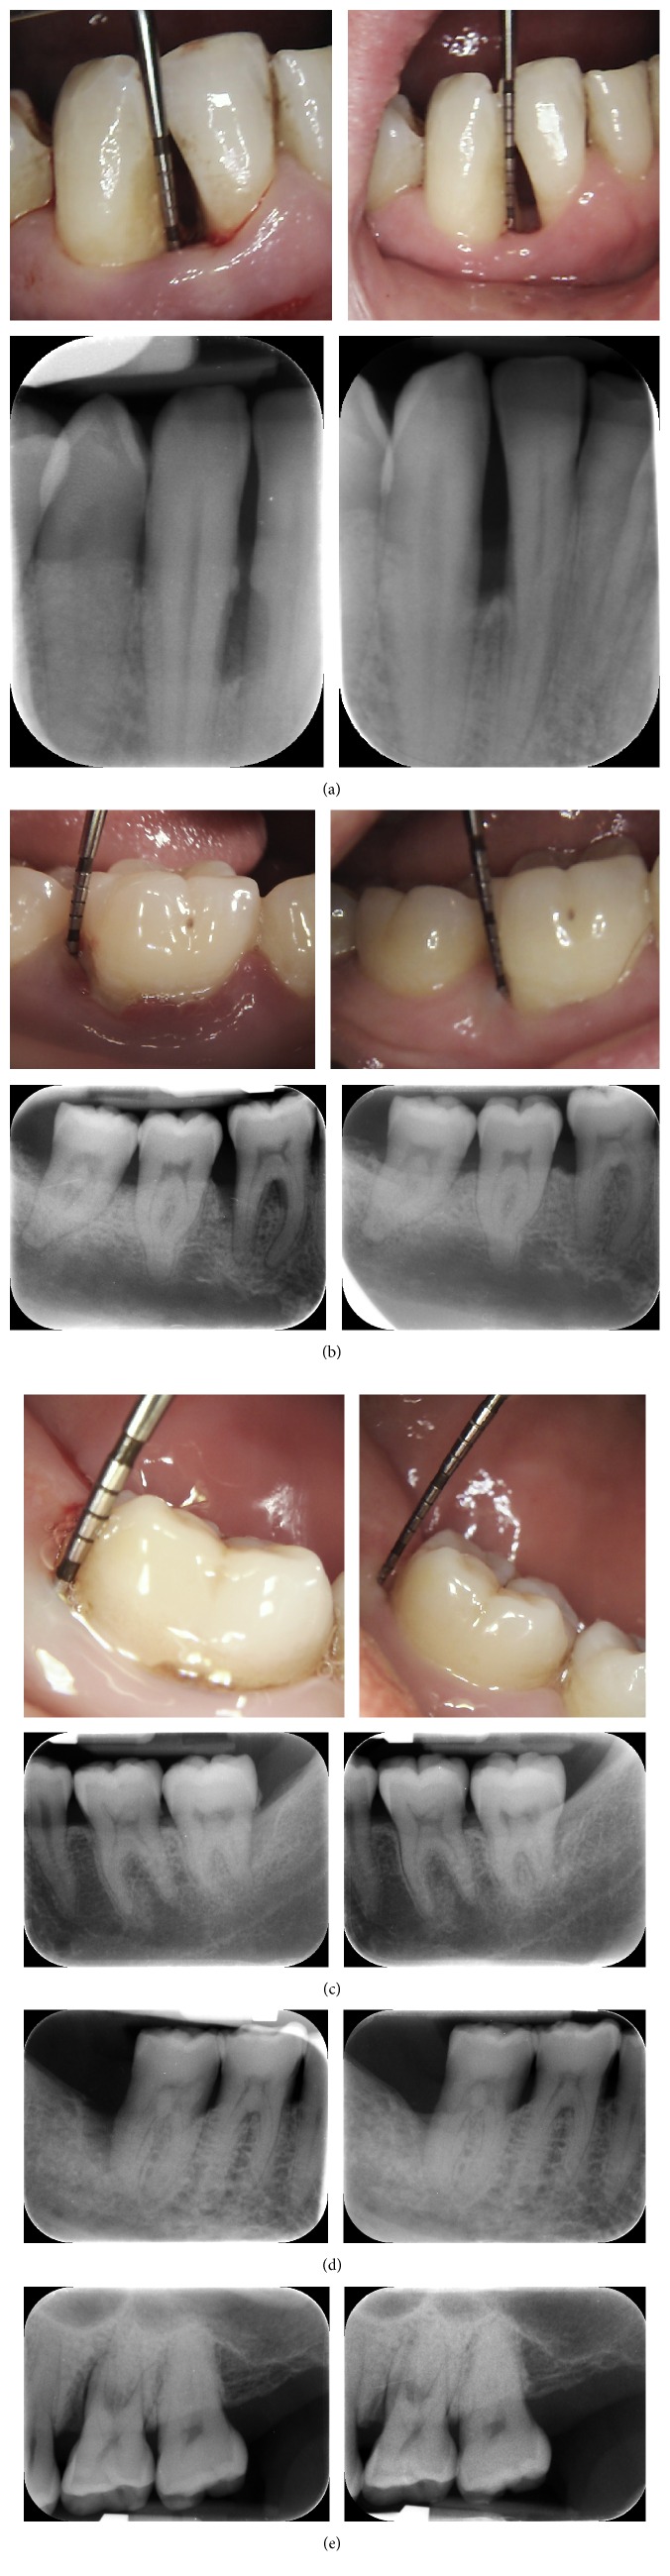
Five cases showing typical results observed. (a) Case 1: 8 mm pocket on the mesial aspect of tooth 42 before treatment, reduced to 3 mm six months later and radiographic bone fill of the defect visible. (b) Case 2: 8 mm defect pre-op associated with an infrabony defect on the distal aspect of 42. The gingivae are inflamed and there is also some recession. Post-operatively, the probing depth is 3 mm, with healthy tissues and minimal recession. The post-op radiograph shows infill of bone distally and in the furcation. (c) Case 3: Pre-op photograph of a 9 mm pocket on the disto-lingual aspect of 37, associated with an infrabony defect as seen on the radiograph. Post-op photograph shows a 2 mm probing depth, with no visible recession having occurred, and the radiograph shows in-fill of bone. (d) Case 4: Pre-op radiograph shows an infrabony defect on the distal aspect of 47 (defect number 28: probing depth was 15 mm). Post-op radiograph shows bone regeneration in the defect (post-op probing depth was 3 mm). (e) Case 5: Defect number 31: pre-op probing depth of 9 mm reduced to 4 mm post-op. There is evidence of radiographic bone fill on the radiograph, but a vertical component of the defect remains, which seems to be the one-wall component of the defect.
